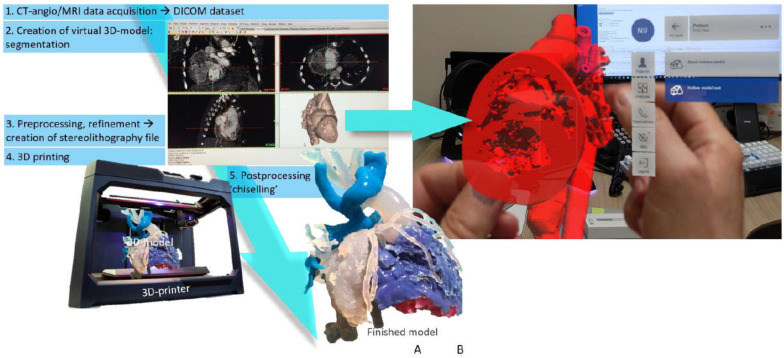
Production phases of 3D modeling for printed prototypes and holograms. (A) Digital raw data acquired from imaging sources (CT angiography, MRI) undergo segmentation to create a 3D virtual model. After preprocessing refinement, the stereolithography file is 3D-printed. Models receive postprocessing treatment. (B) 3D virtual model as a hologram can also be imported into virtual reality, where it can be fully rotated and entered.
